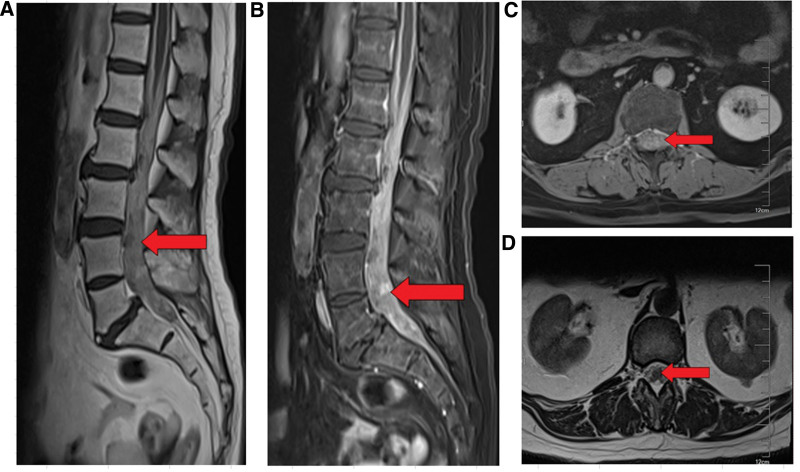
MRI of the lumbar spine. (A–D) Lumbar spine MRI. MRI = magnetic resonance imaging.
